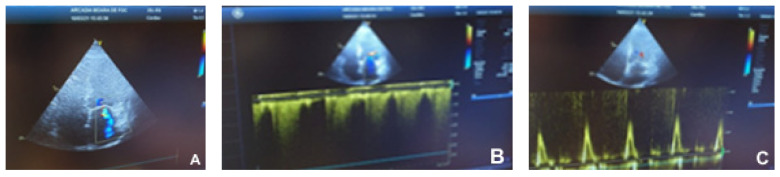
Dilated cardiomyopathy echocardiogram. (A)—Severe mitral regurgitation (apical four-chamber); (B)—tricuspid envelope continuous Doppler; (C)—trans-mitral pattern that is suggestive of atrial fibrillation.
